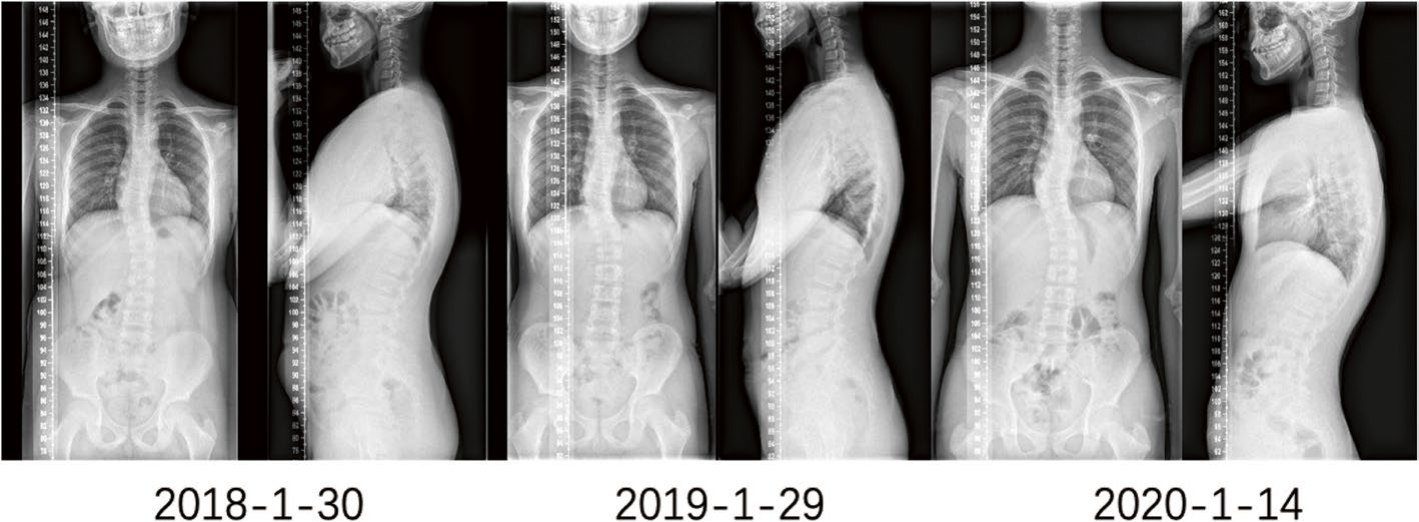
A patient chose conservative treatment and underwent three follow-up visits with a one-year interval, and X-ray images of the entire spine were displayed in both the anterior and lateral positions
